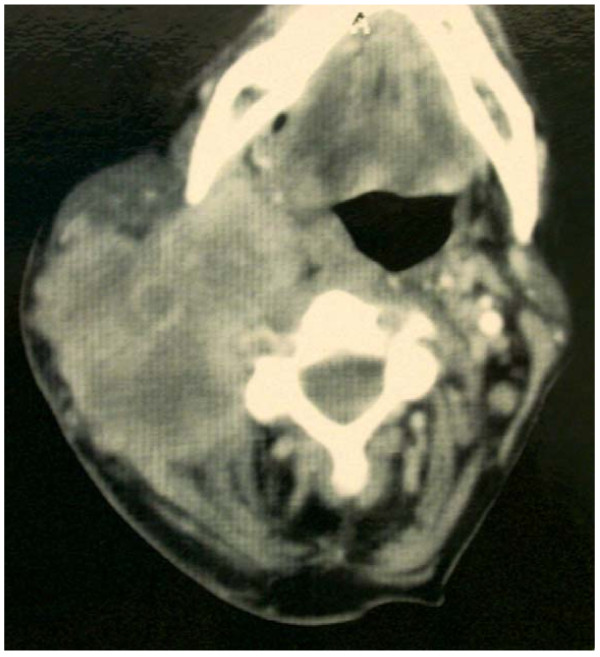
showing axial CT scan of necrotic right cervical lymphadenopathy.
